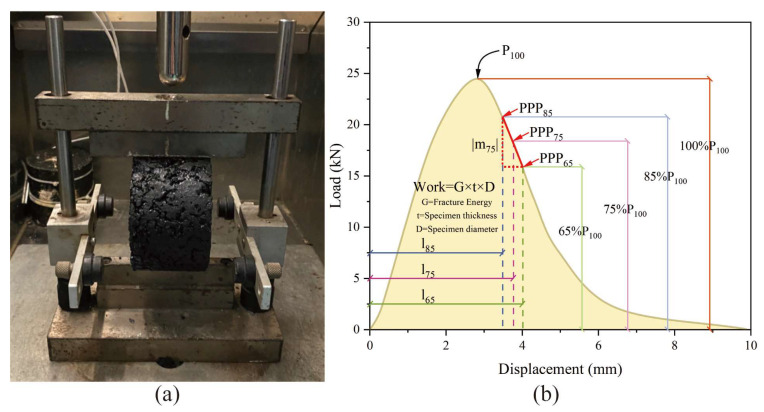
IDEAL-CT test: (a) test device and (b) typical load–displacement curve.
